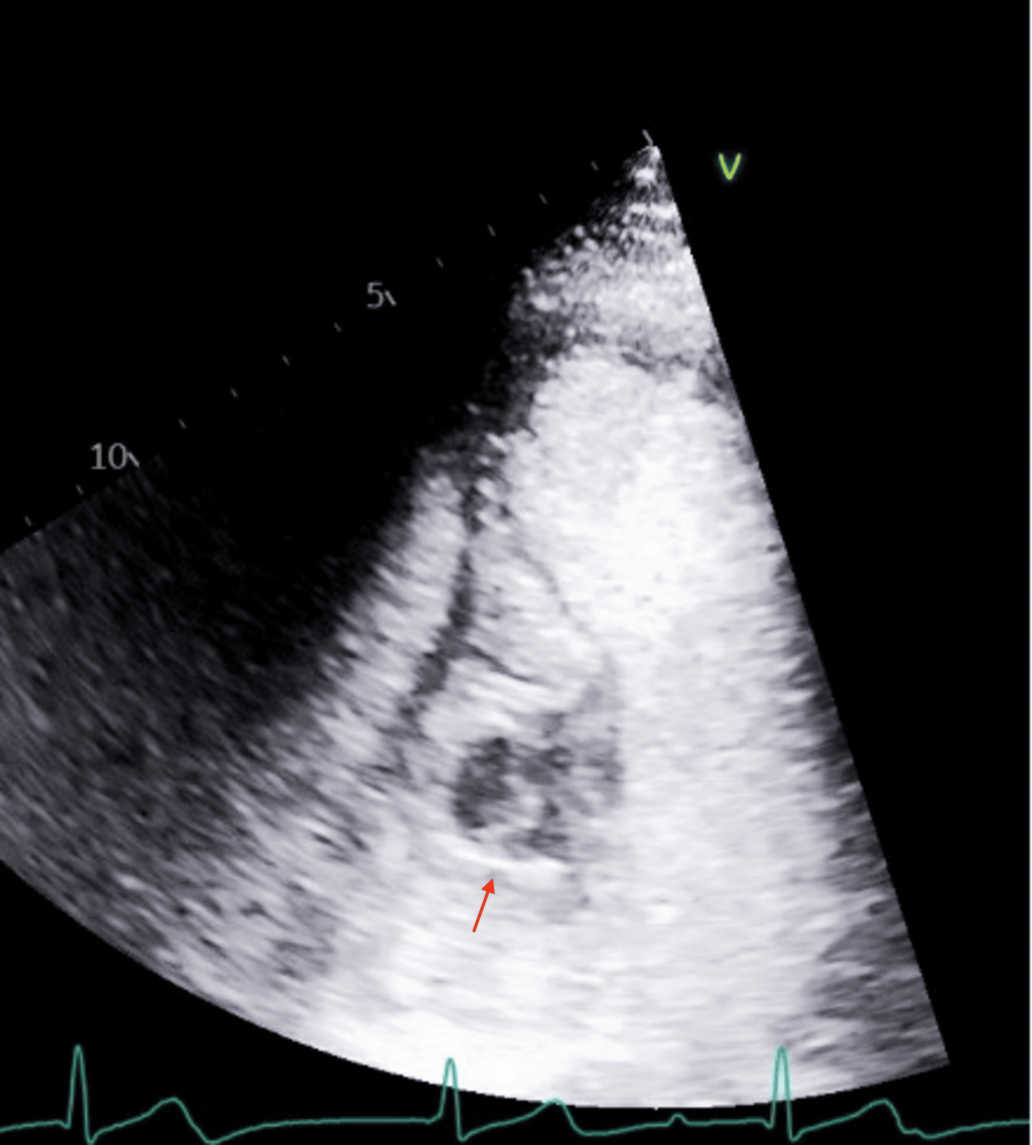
Apical four-chamber view on TTE with ultrasound-enhancing agent (UEA) showing a complex mass with a central region of non-enhancement and heterogeneous contrast uptake, suggesting a cystic component TTE: transthoracic echocardiography
